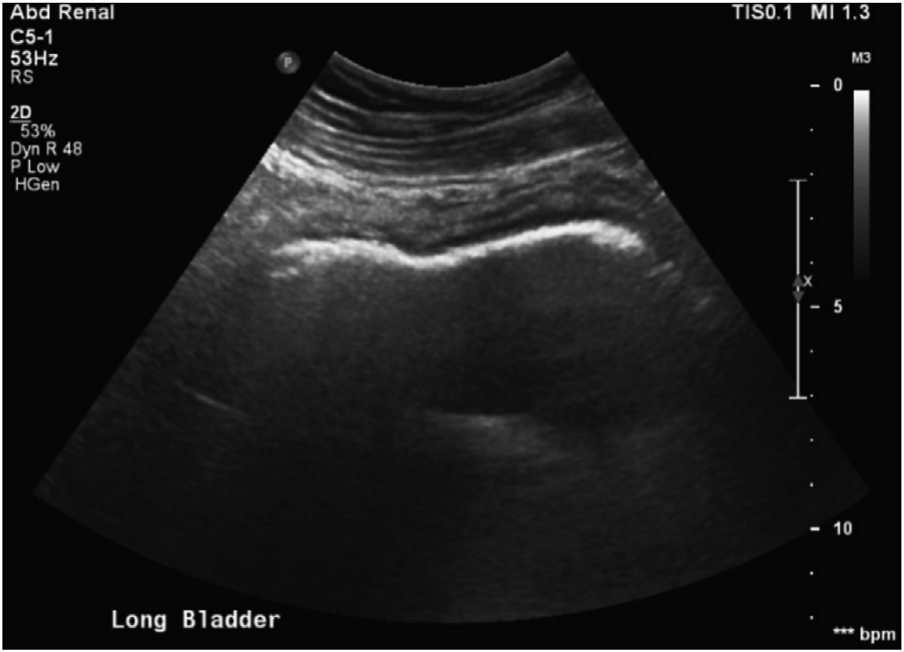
Bladder ultrasound demonstrating echogenic gas in the bladder wall.
